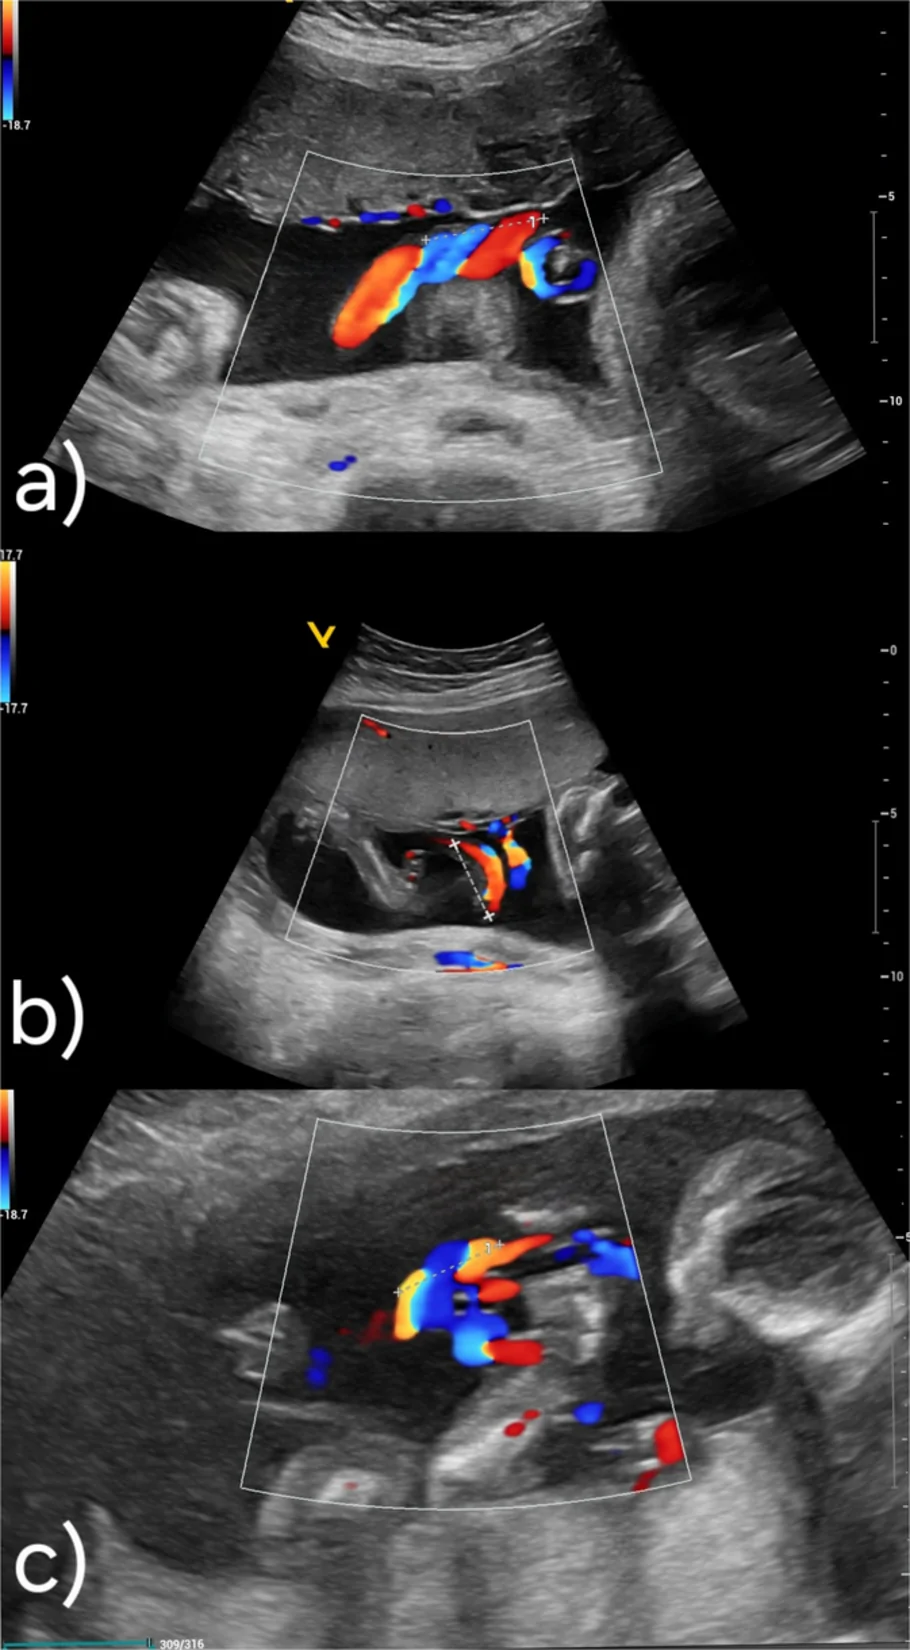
Representative color Doppler ultrasound images demonstrating the technique for antenatal assessment of the umbilical cordcoiling index: (a) normocoiled, (b) hypocoiled, and (c) hypercoiled umbilical cords.
